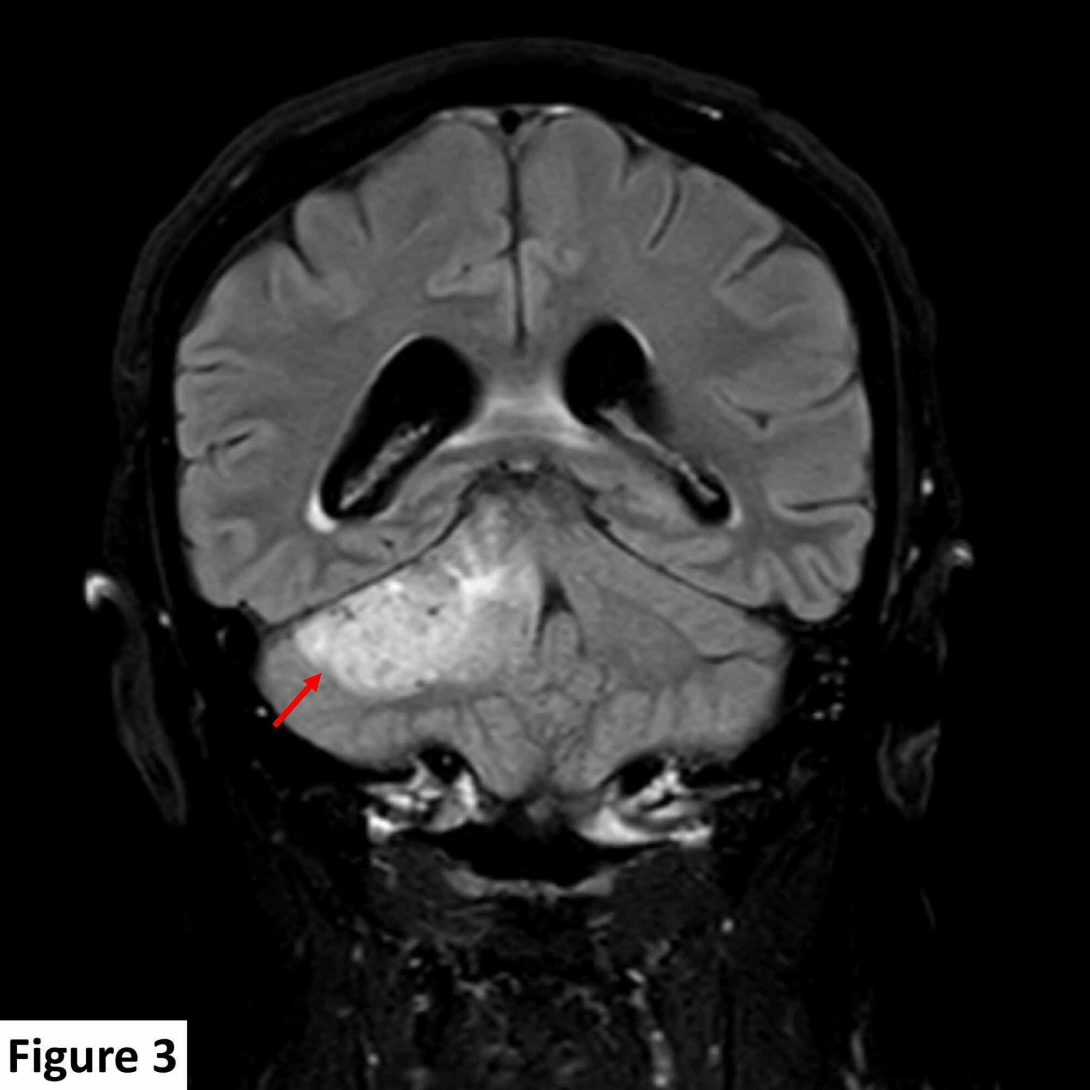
MRI scan at presentation. Coronal T2 FLAIR image shows a right cerebellopontine angle hyperintense extra-axial dural-based lesion (red arrow). MRI, magnetic resonance imaging; FLAIR, fluid attenuated inversion recovery
